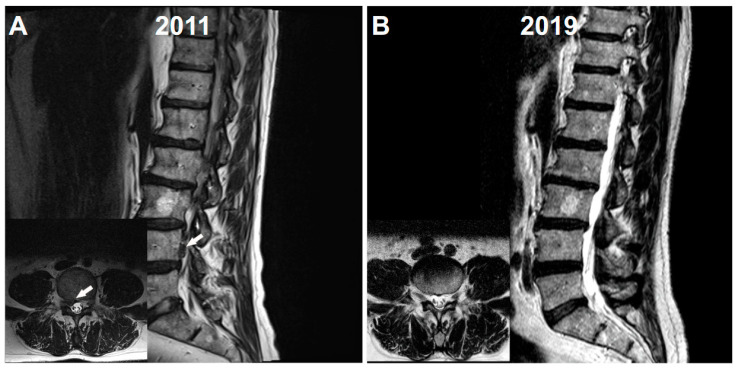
(A) T2 weighted MRI imaging from 2011 shows a herniation of the L4-5 disc. A cranially migrated sequestered disc fragment is marked by an arrow. The insert shows corresponding transversal MRI imaging. The herniation compresses the right L4 root (marked by an arrow). (B) Corresponding T2 weighted MRI sagittal and transversal (insert) images from 2019. No herniation of the L4-5 disc can be observed anymore. The right L4 root is not affected (see insert).
